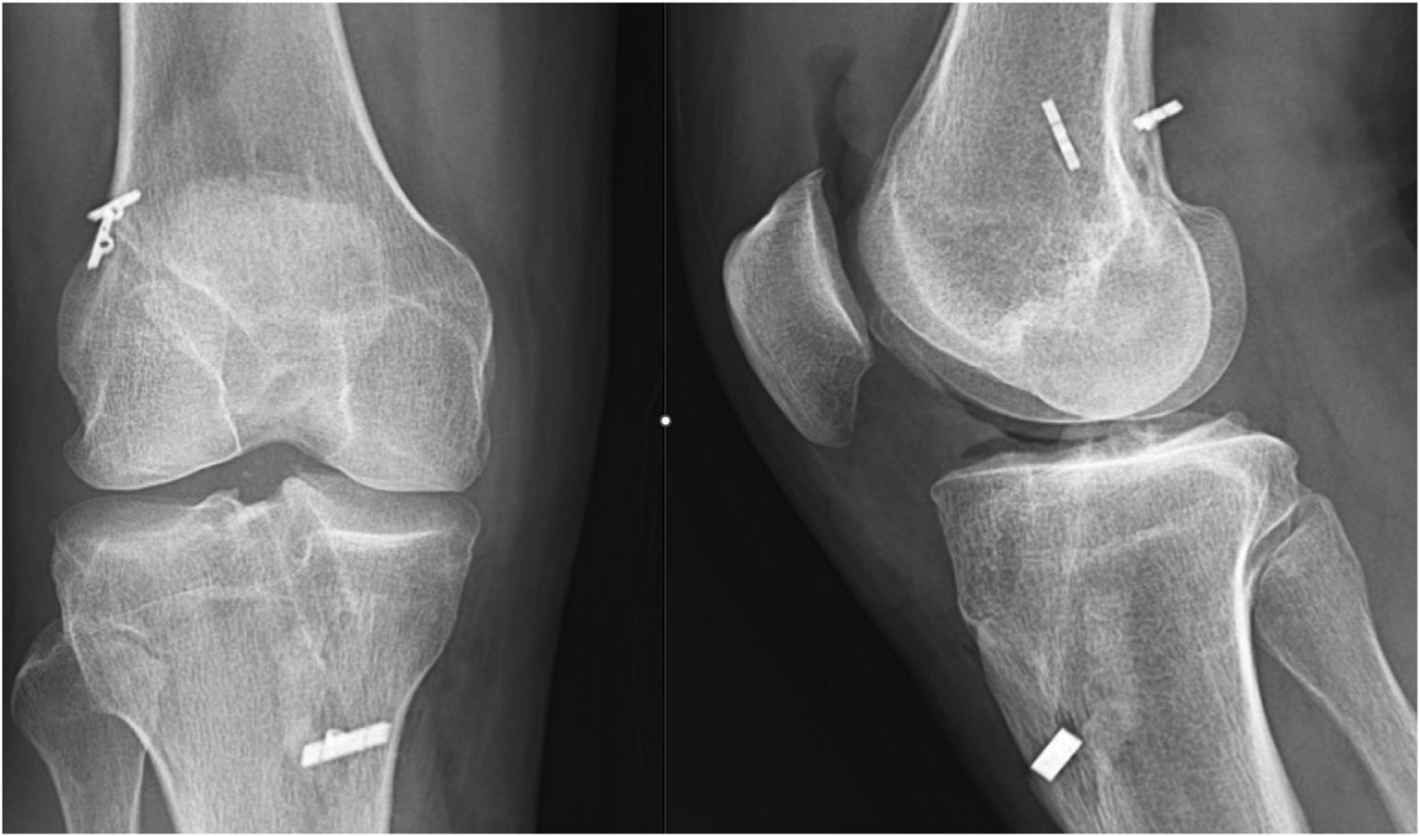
A postoperative radiograph of the right knee showcasing the results of a single-stage anterior cruciate ligament reconstruction revision with double suspensory fixation and simultaneous tunnel grafting.
